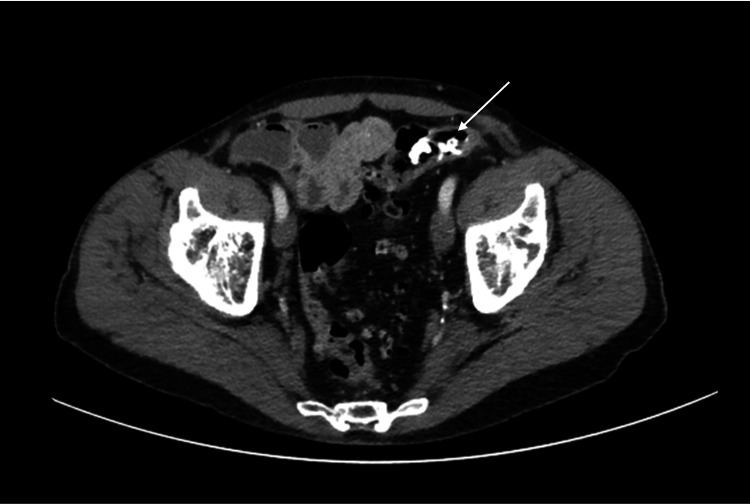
Axial CTAP showing calcified fragments at the descending/sigmoid colon junction (arrowed). CTAP = Computed tomography of abdomen and pelvis
